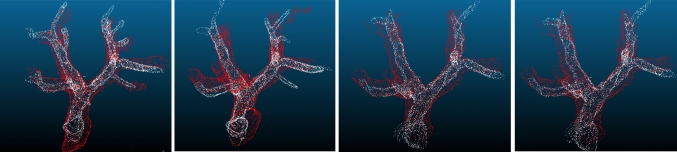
Partial registration results. White point means US-based, and red point means CT-based. The images show the samples among plots in Fig. 11
